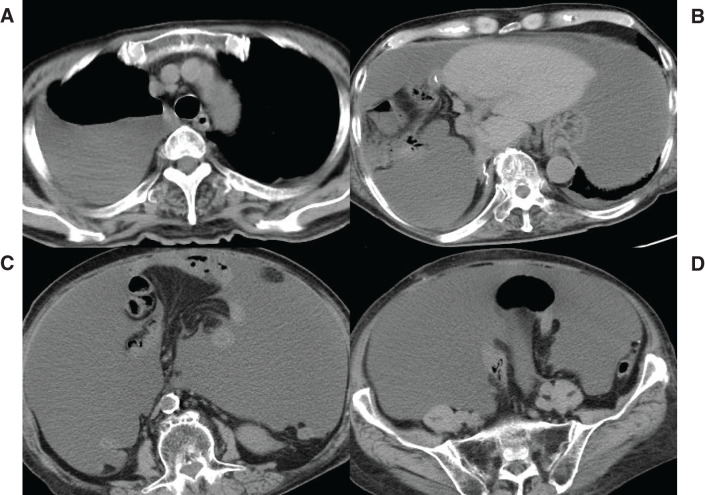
CT findings at readmission. Massive pleural effusion was present, mainly in the right hemithorax (A). Ascites surrounded the remnant liver (B) and much of the abdomen (C), extending to the pelvis (D).
